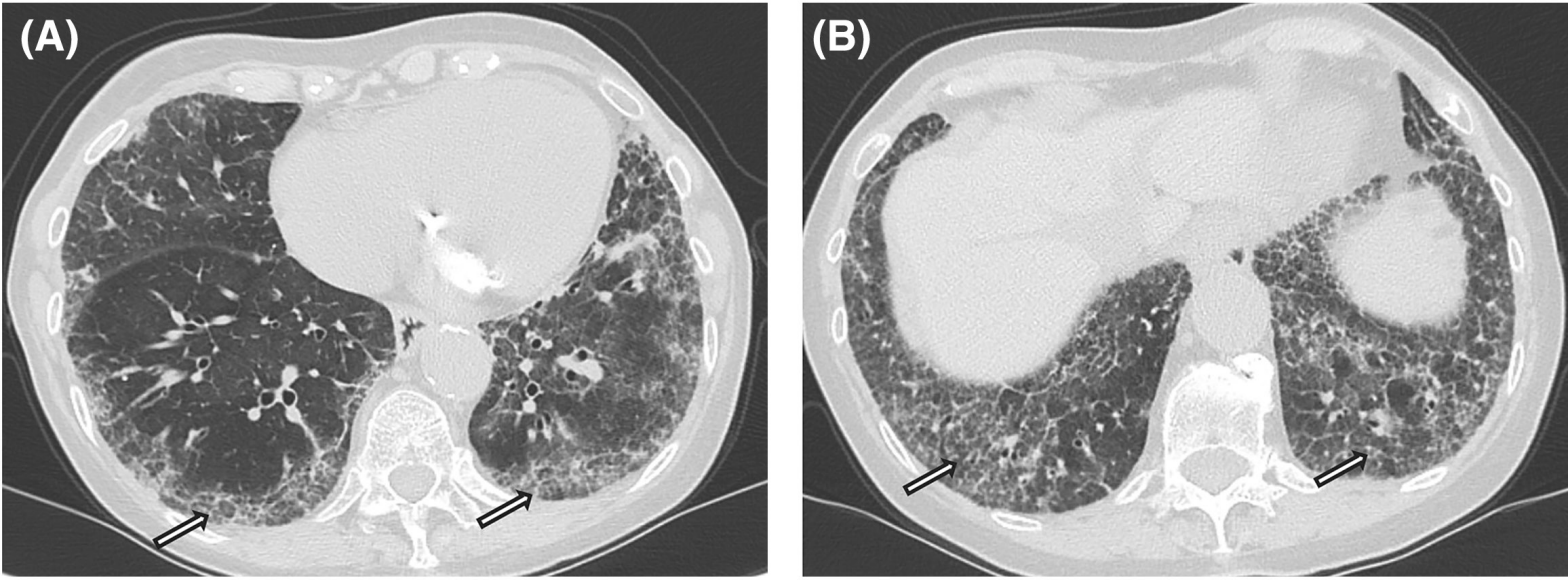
(a) Lung HRCT, April 2023, showed new lung alterations, sustained by ground glass opacities, reticulations and crazy paving appearance (white arrows). (b) Basal and peripheral distribution is predominant.
